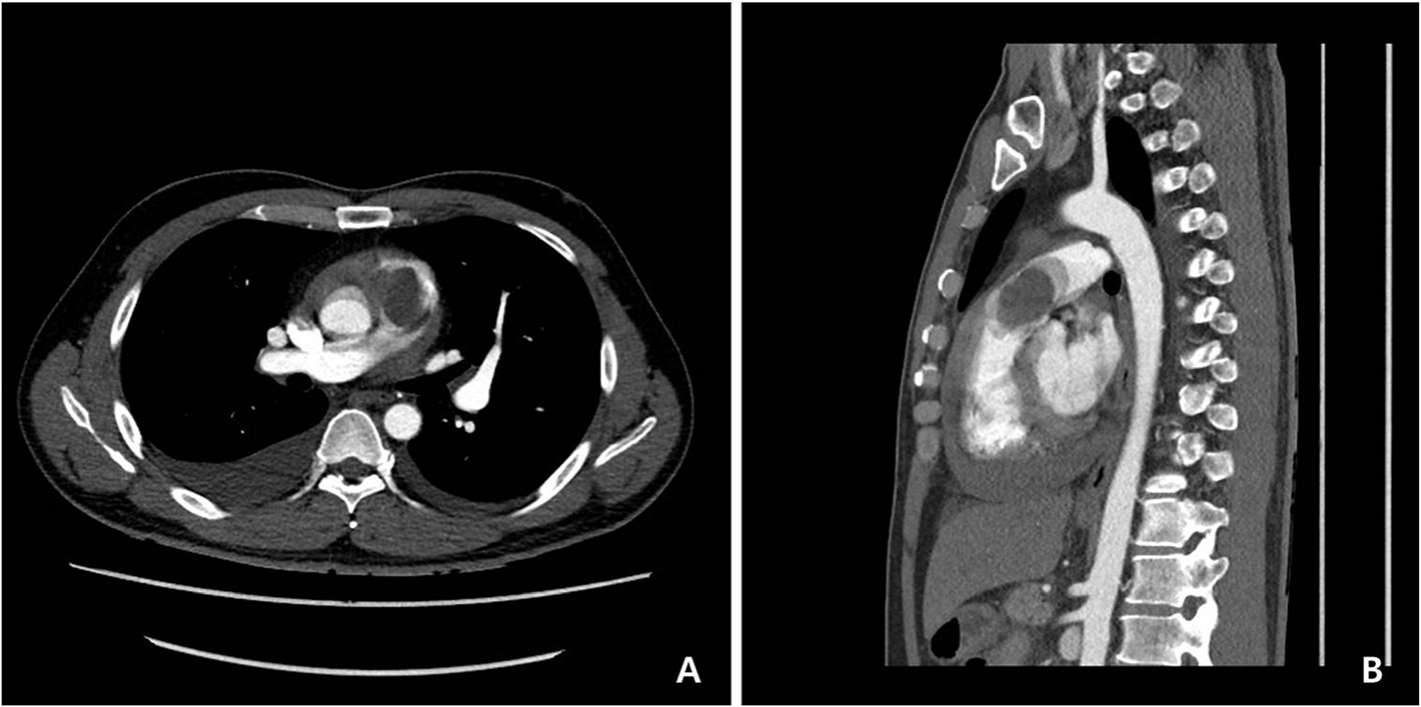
CT axial (a) and sagittal (b) images showing a mass completely obstructing the right ventricular outflow tract
